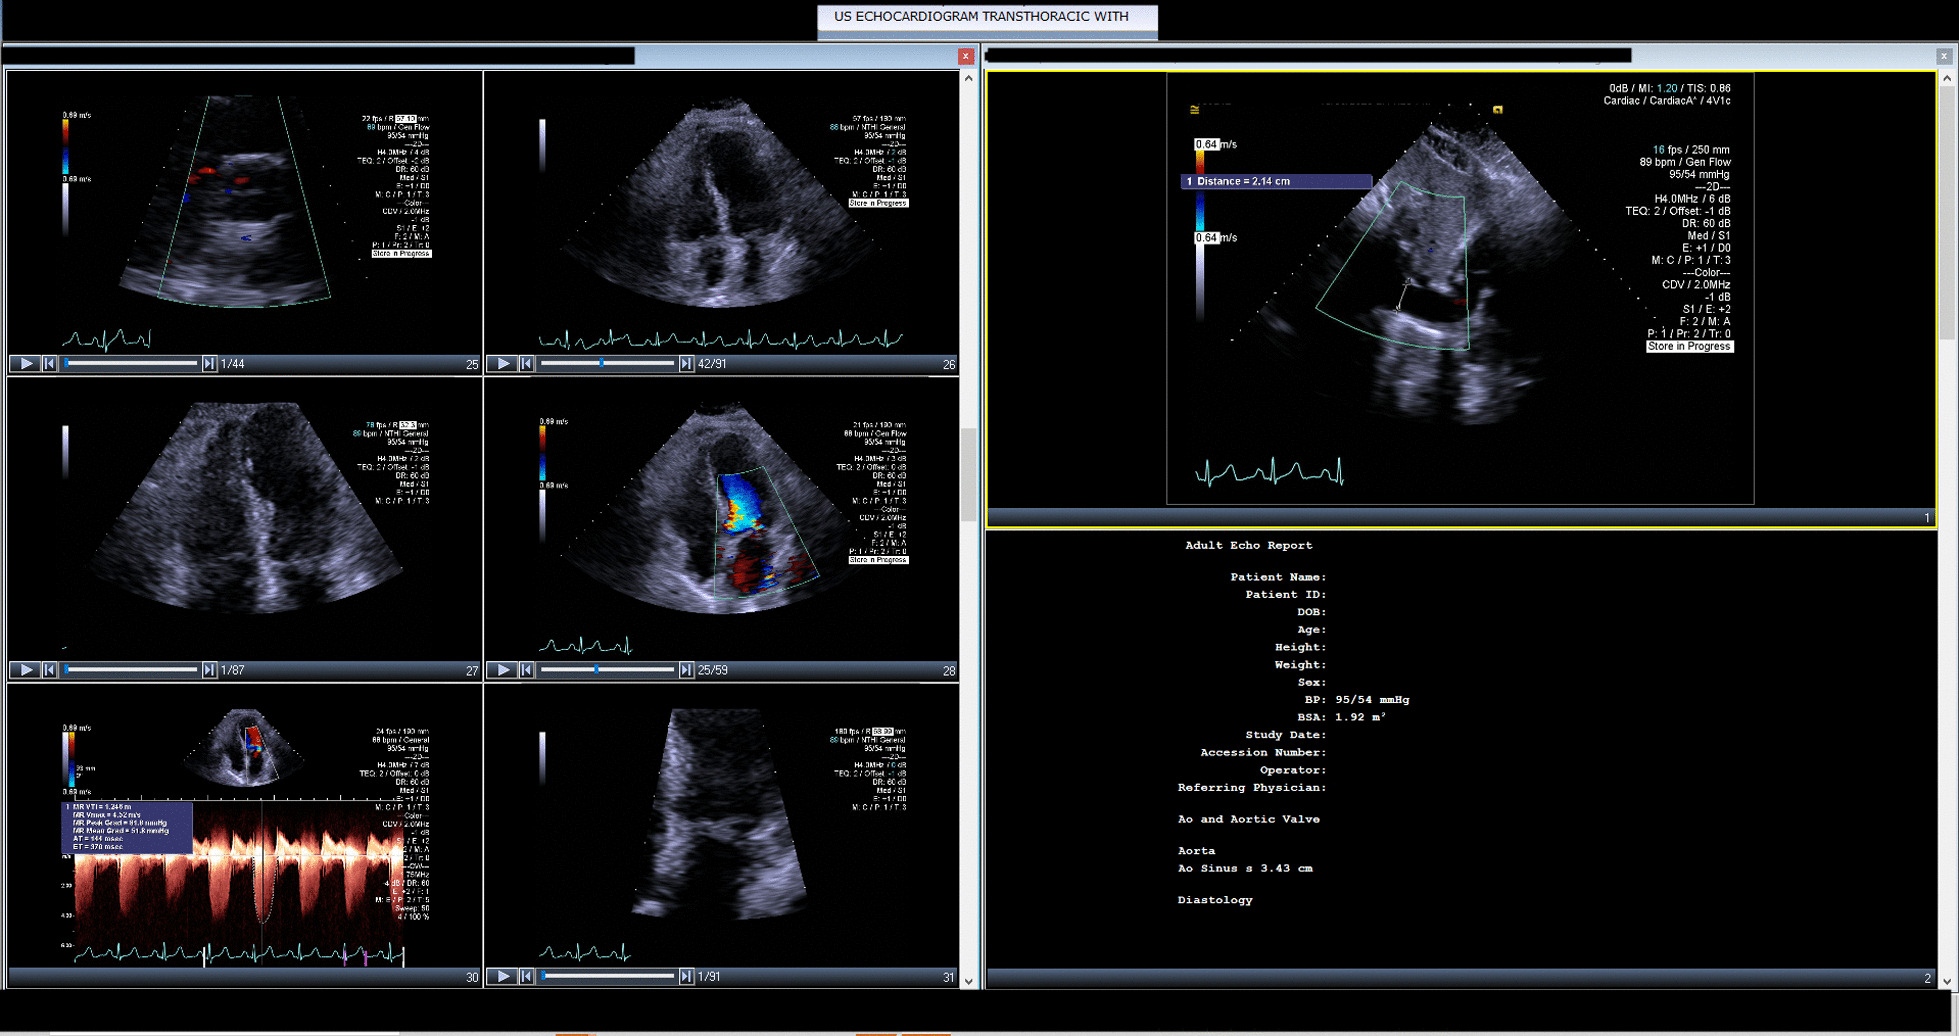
Transthoracic echocardiography revealed at least moderate mitral regurgitation
